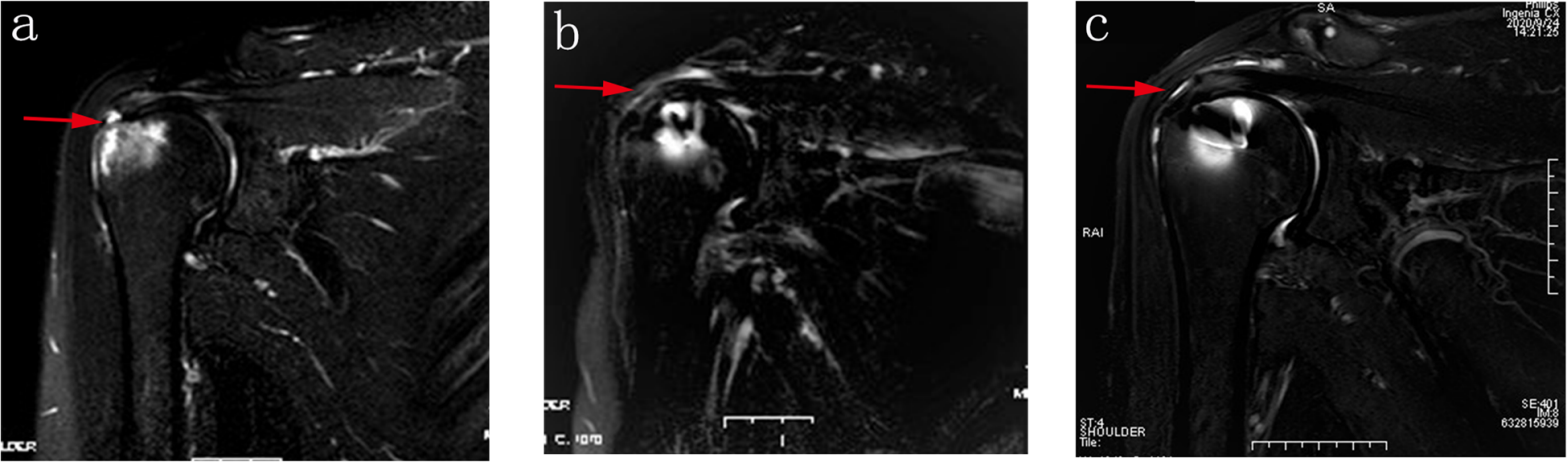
MRI for the rotator cuff. a Preoperative imaging revealed the torn supraspinatus tendon (red arrow). b Three-month postoperative imaging shows the footprint regeneration (red arrow). c Six-month postoperative imaging shows more growth in the footprint (red arrow)
